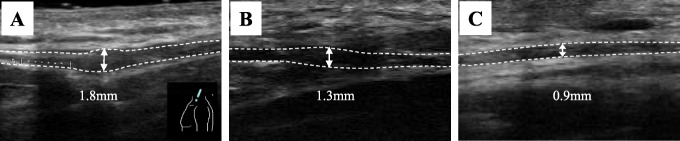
US examination findings. a Ultrasonography was performed using a Canon Aplio i800 with a 24-MHz linear probe. Preoperatively, the SAN was swollen (1.8-mm width) at the central sternocleidomastoid muscle level within the posterior cervical triangle, but nerve continuity was preserved. The diameter of the SAN gradually improved from 1.8 mm preoperatively to 1.3 mm at 3 months (b) and 0.9 mm at 6 months (c), at which time there was no swollen area
